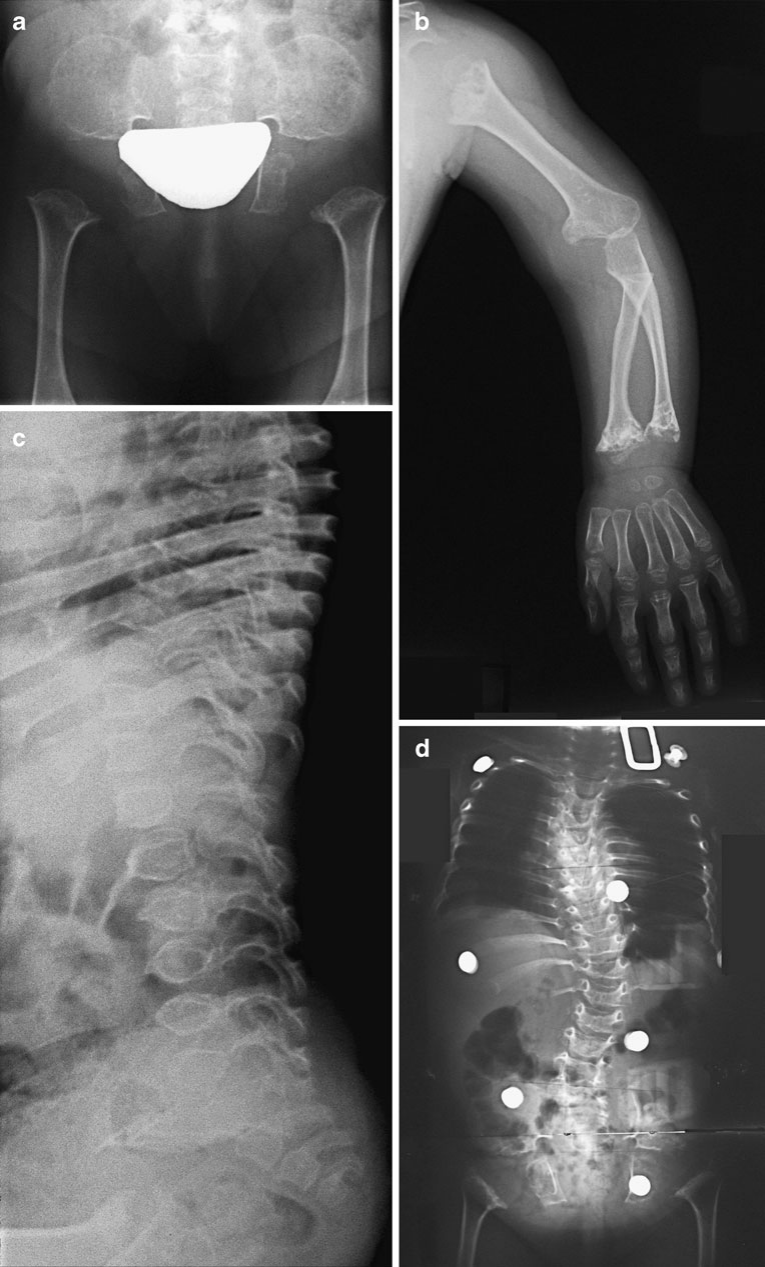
a-d Patient’s radiographs. a. Pelvis of the 2-year-old patient. There are round iliac wings, horizontal acetabular roof, short sciatic notches, broad ischia, very short and broad femoral neck and absent capital femoral ossification centres. b. Left upper extremity radiograph of the 3-year-old patient shows short humerus, marked metaphyseal involvement of the proximal humerus as well as distal radius and ulna and delayed carpal bone age. c. Lateral view of spine taken at the age 3 years demonstrates ovoid vertebral bodies with anterior tongue-like protrusion. d. a-p view of spine at the age 4 years reveals marked thoraco-lumbar scoliosis convex to the left
